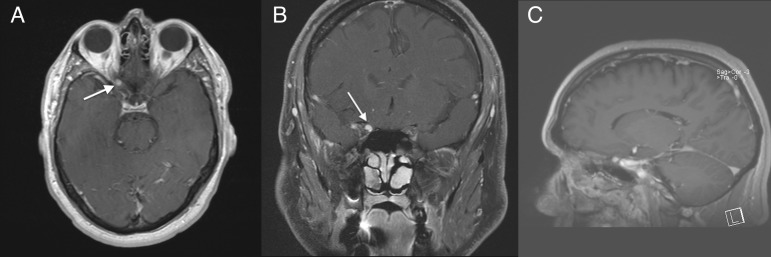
Preoperative T1-weighted MRI with contrast Axial view showing a small enhancing lesion along the optic nerve at the orbital apex pointed out by the white arrow (A). Coronal (B) and sagittal (C) views again showing the enhancing orbital mass.
